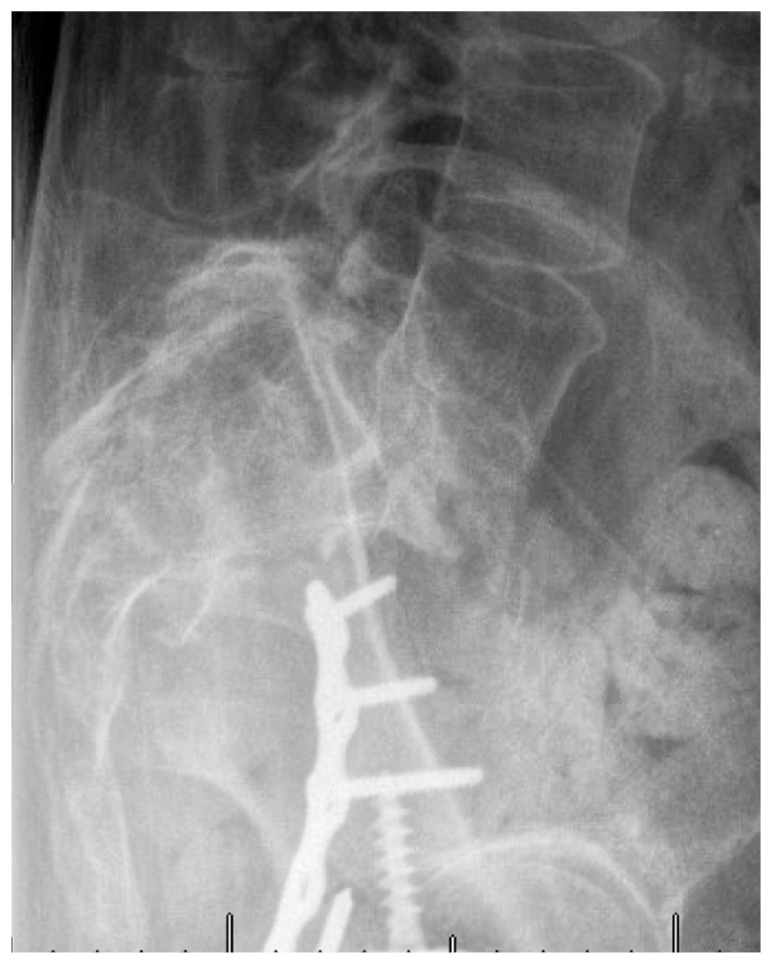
Pre-operative lateral x-ray demonstrating L5-S1 spondylolisthesis and sacral kyphosis.
